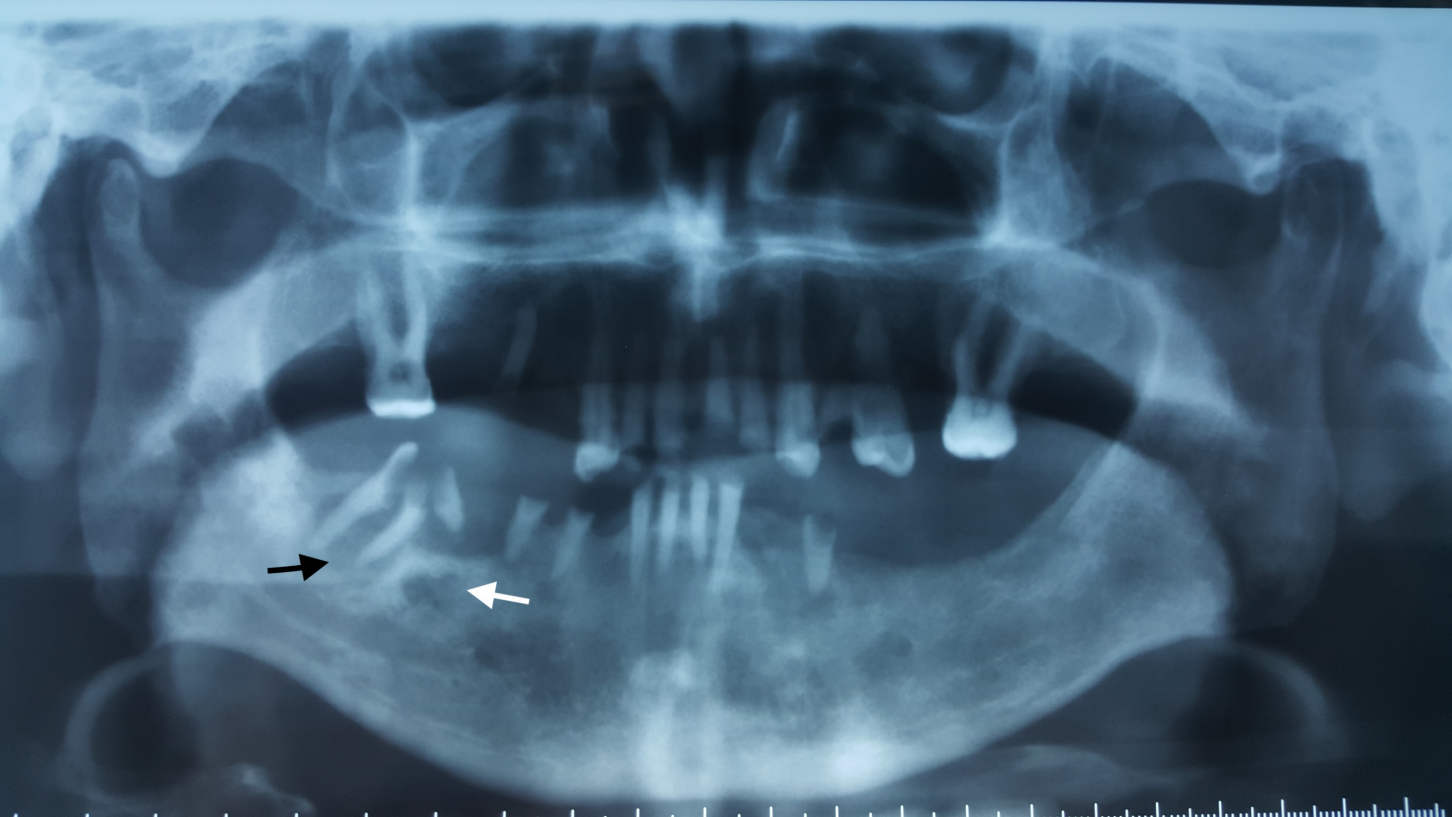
Orthopantomogram revealed two radiolucent lesions associated with the roots of 46 (white arrow) and 47 (black arrow)
